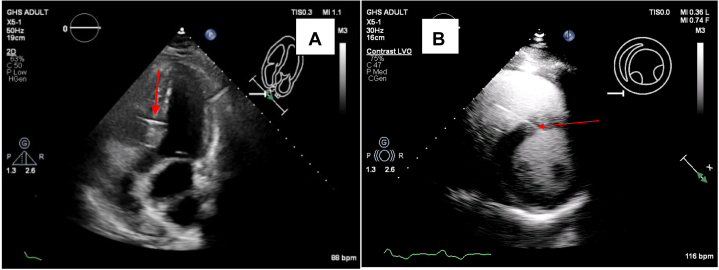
Echocardiogram at 3 Months After Upgrade (A) Long-axis apical and (B) short-axis views of the patient's heart on echocardiogram. The pacing lead (red arrows) is buried securely in the left ventricular septal subendocardium.
